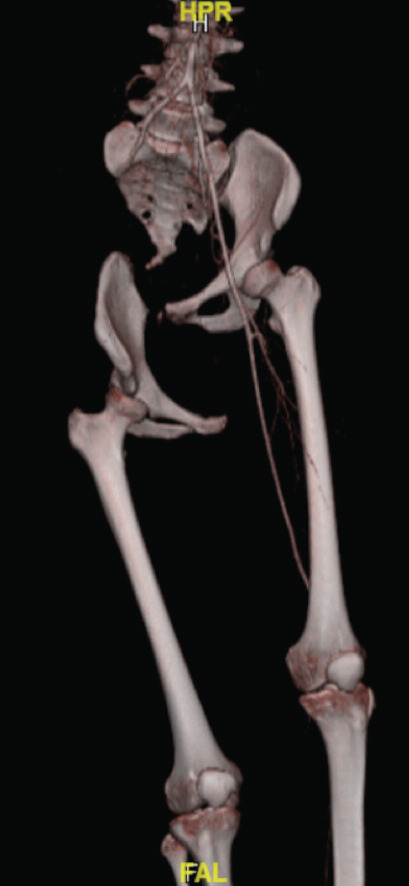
3D reconstructed Computer tomography angiogram (CTA) showed loss of arterial contrast flow at proximal right external iliac artery and right hemipelvis was sheared inferiorly.
